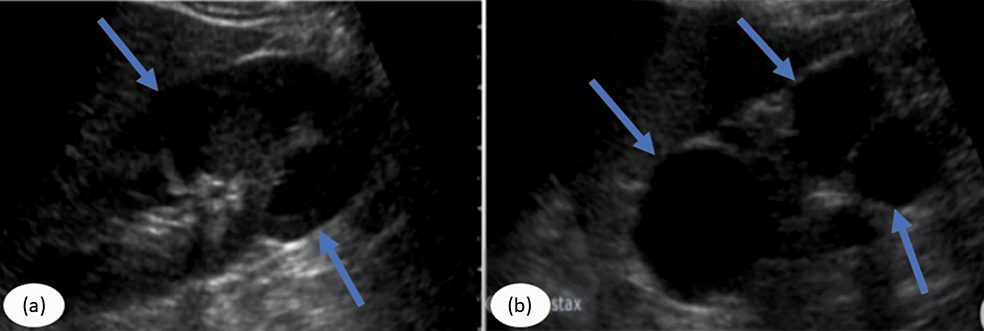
Ultrasound KUB showing cysts of various sizes on each pole of the left kidney (a,b). KUB: kidney, ureter, bladder.
